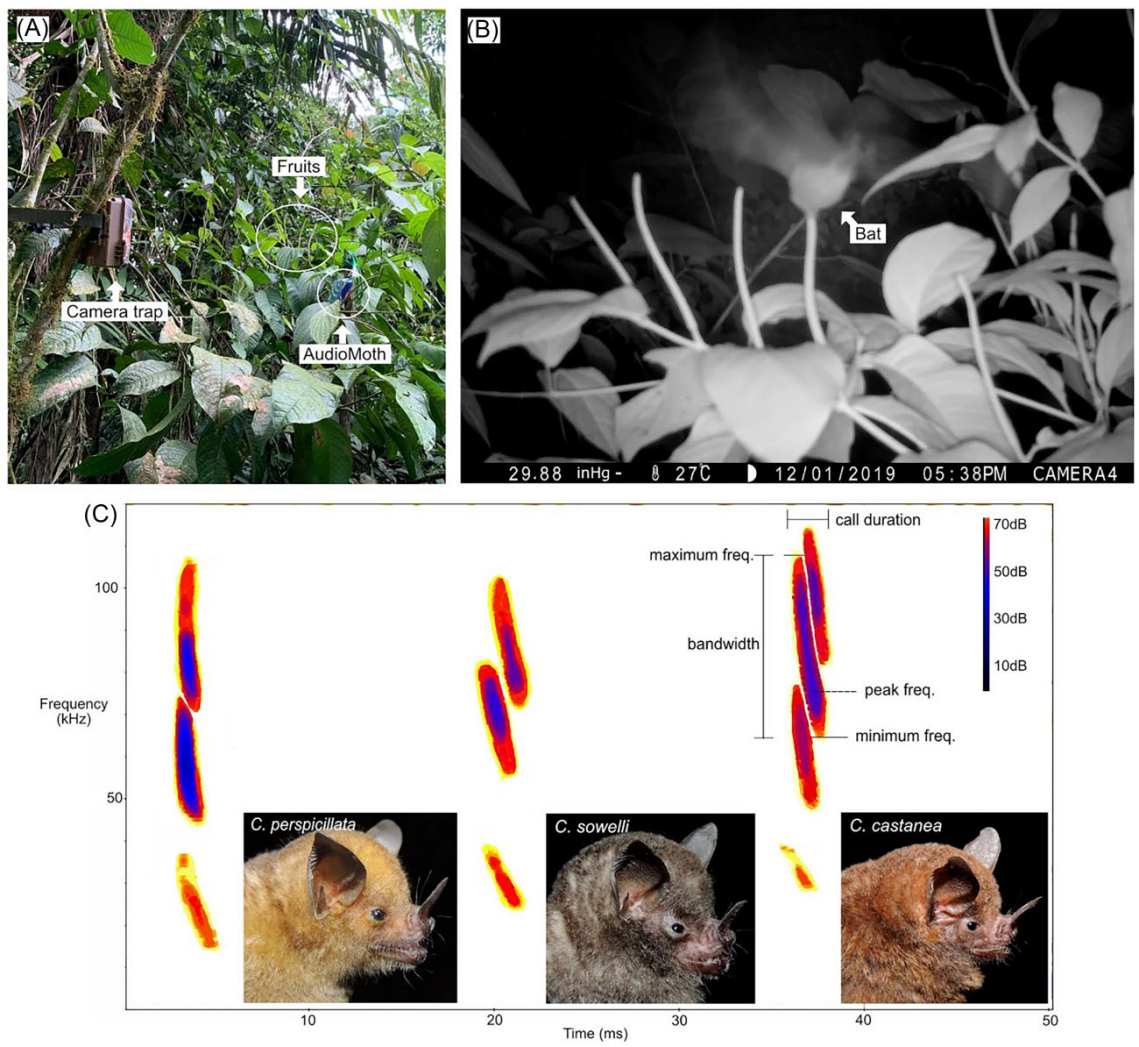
Experimental setup including camera trap and ultrasonic acoustic recorder (AudioMoth) deployed at a *Piper sancti-felicis* plant in the field (A), a video frame showing a bat collecting a fruit at the same plant (B; video available as a supplementary file [Supplementary Video 1]), and reference echolocation calls for *Carollia perspicillata, C. sowelli*, and *C. castanea* (C; spectrograms generated in BatSound v4.4). Analysis of acoustic data was performed using the parameters marked in the spectrogram (call duration, peak frequency, minimum frequency, maximum frequency, and bandwidth of the main harmonic; see Table S2). *Carollia* photos credit: David Villalobos Chaves.
